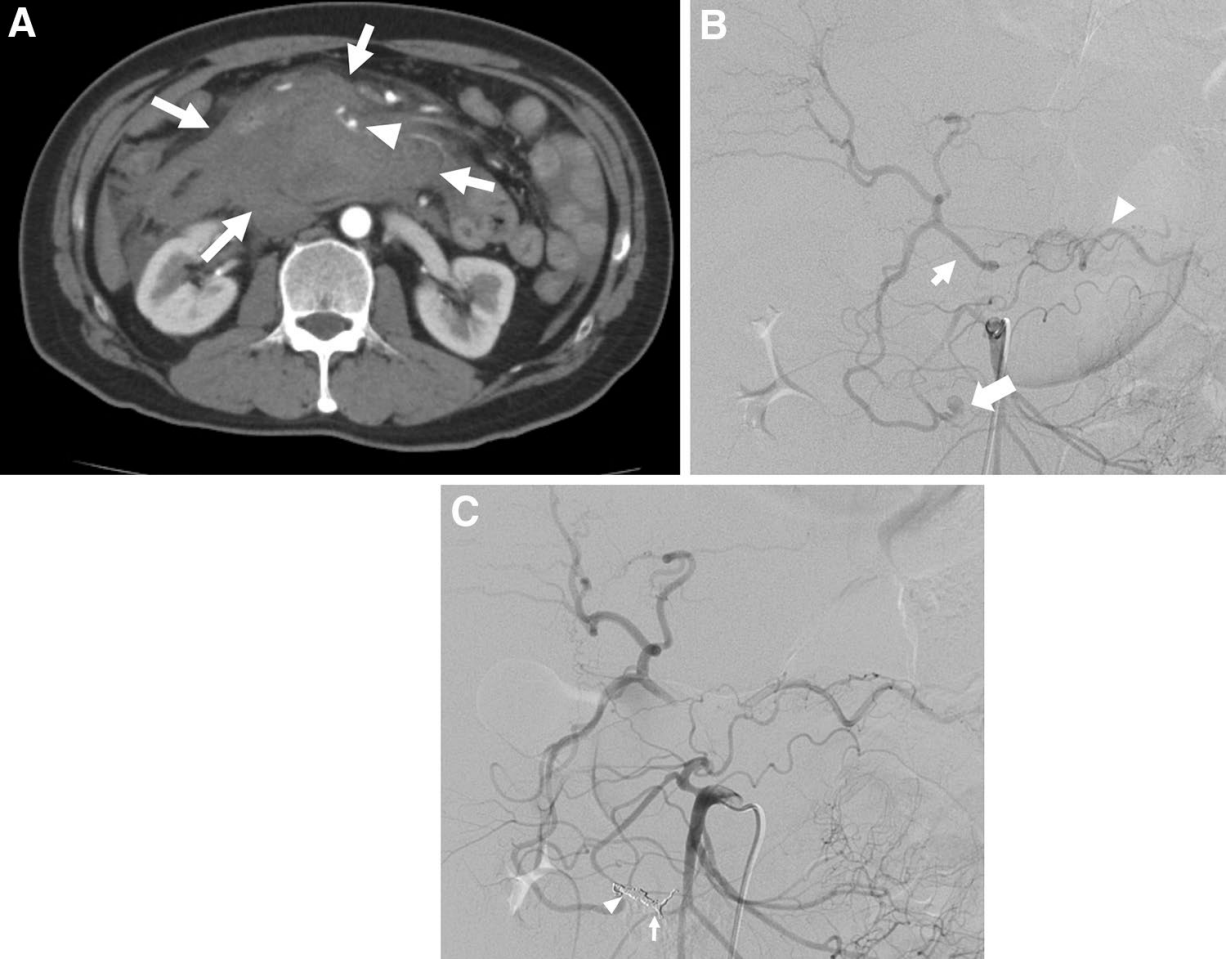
Pseudoaneurysm in a branch of the pancreaticoduodenal arcade. a Arterial phase contrast-enhanced CT scan shows a massive retroperitoneal hematoma (arrow) surrounding the pancreatic head. A pseudoaneurysm (arrowhead) is visualized in the hematoma. b Selective superior mesenteric digital subtraction angiography shows visualization of the hepatic (arrow) and splenic arteries (arrowhead) via collateral circulation, suggesting severe stenosis or occlusion of the celiac artery. The ruptured aneurysm (large arrow) can be seen in the branch of pancreaticoduodenal arcade. c Embolization using metallic coils of the distal site of the aneurysm (arrow) and the proximal site of the aneurysm (arrowhead) resulted in complete embolization of the pseudoaneurysm with an isolation technique
